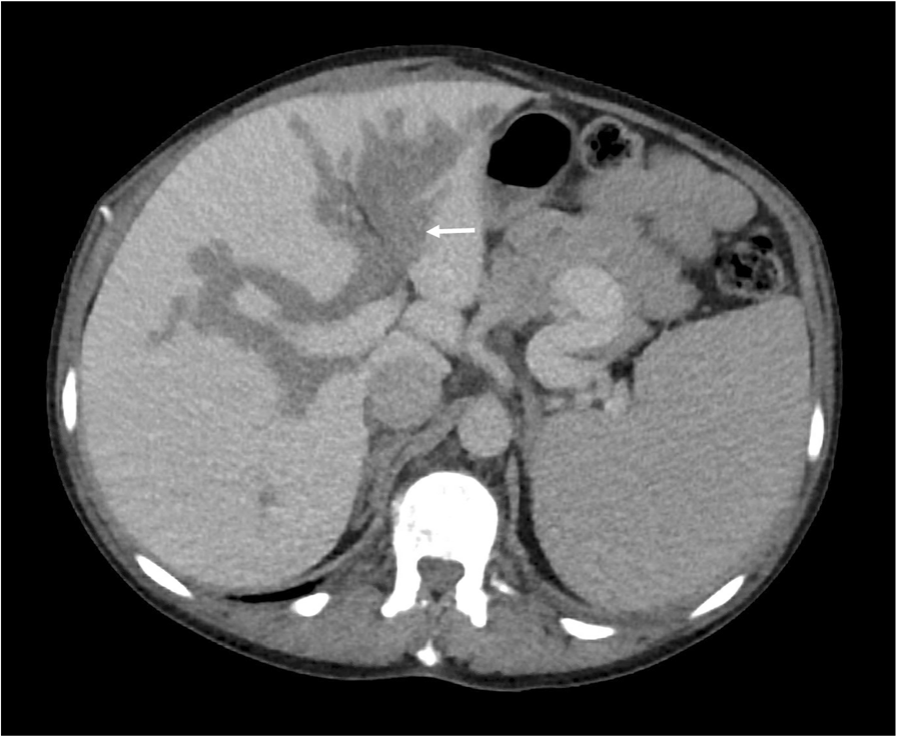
Axial CT demonstrating potential sites of extramedullary haematopoiesis (EMH) in the body. The commonest sites of involvement are the liver and spleen which manifest as hepatosplenomegaly. Less commonly, EMH may present in the periportal region (white arrows) and may be indistinguishable from periportal oedema. Clues to differentiating periportal EMH from periportal oedema include a more lobular appearance to EMH and soft tissue attenuation, whereas periportal oedema is generally less lobular and may have fluid attenuation
